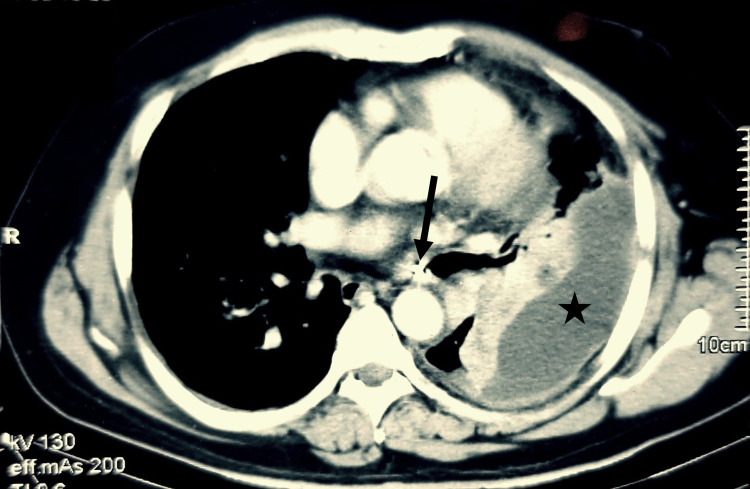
Axial CT scan (mediastinal window) performed one year previously. The metallic density with streak artifacts noted within the left main bronchus (black arrow). Left-sided empyema (black star) with adjacent left lower lobe atelectasis. CT: computed tomography
